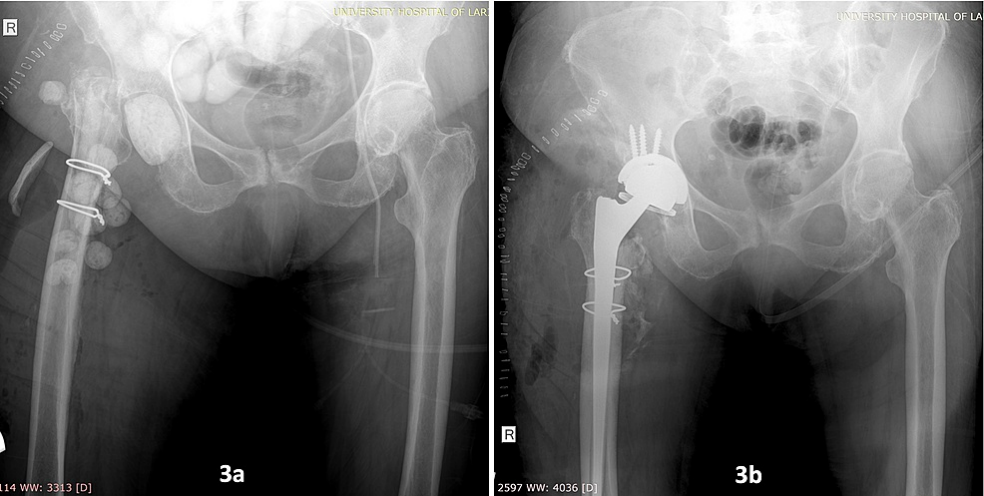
Postoperative X-rays. X-ray after first-stage surgical debridement and antibiotic cement spacers application (3a) and after second-stage reimplantation with constrained total hip arthroplasty (3b).
